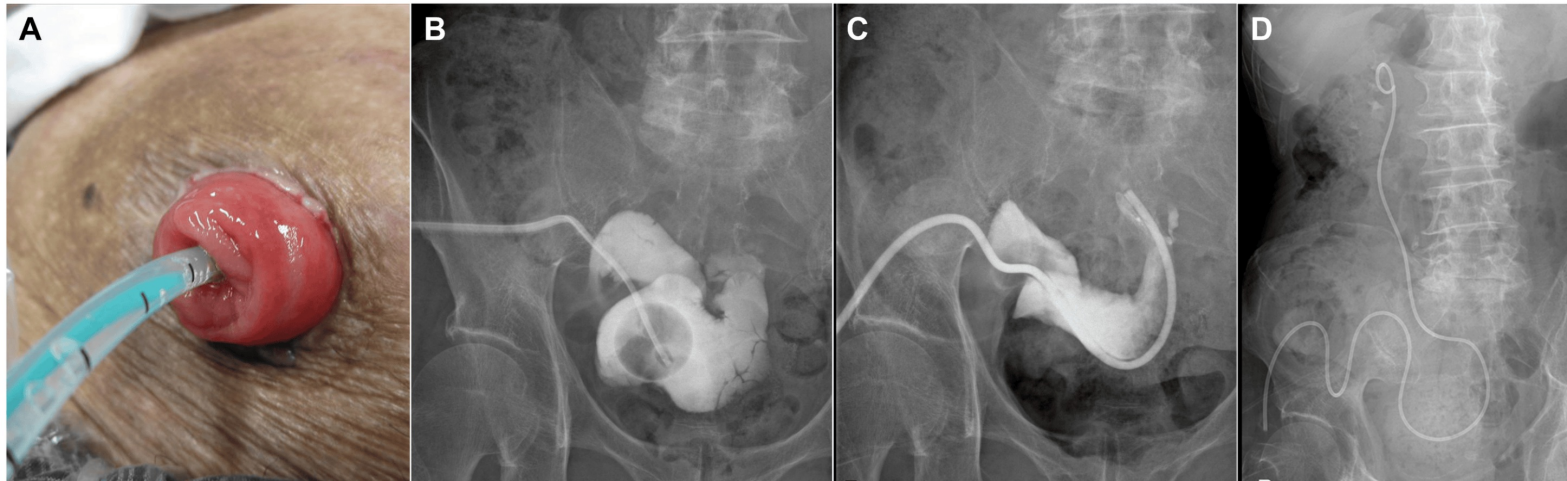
History of catheter management for recurrent obstruction (A) Appearance of the ileal conduit stoma with an indwelling renal pelvis balloon catheter. (B) Abdominal radiograph showing the indwelling renal pelvis balloon catheter. (C) Abdominal radiograph showing a Nelaton catheter placed in the ileal conduit. (D) Abdominal radiograph showing a single-J (SJ) catheter placed in the right renal pelvis.
